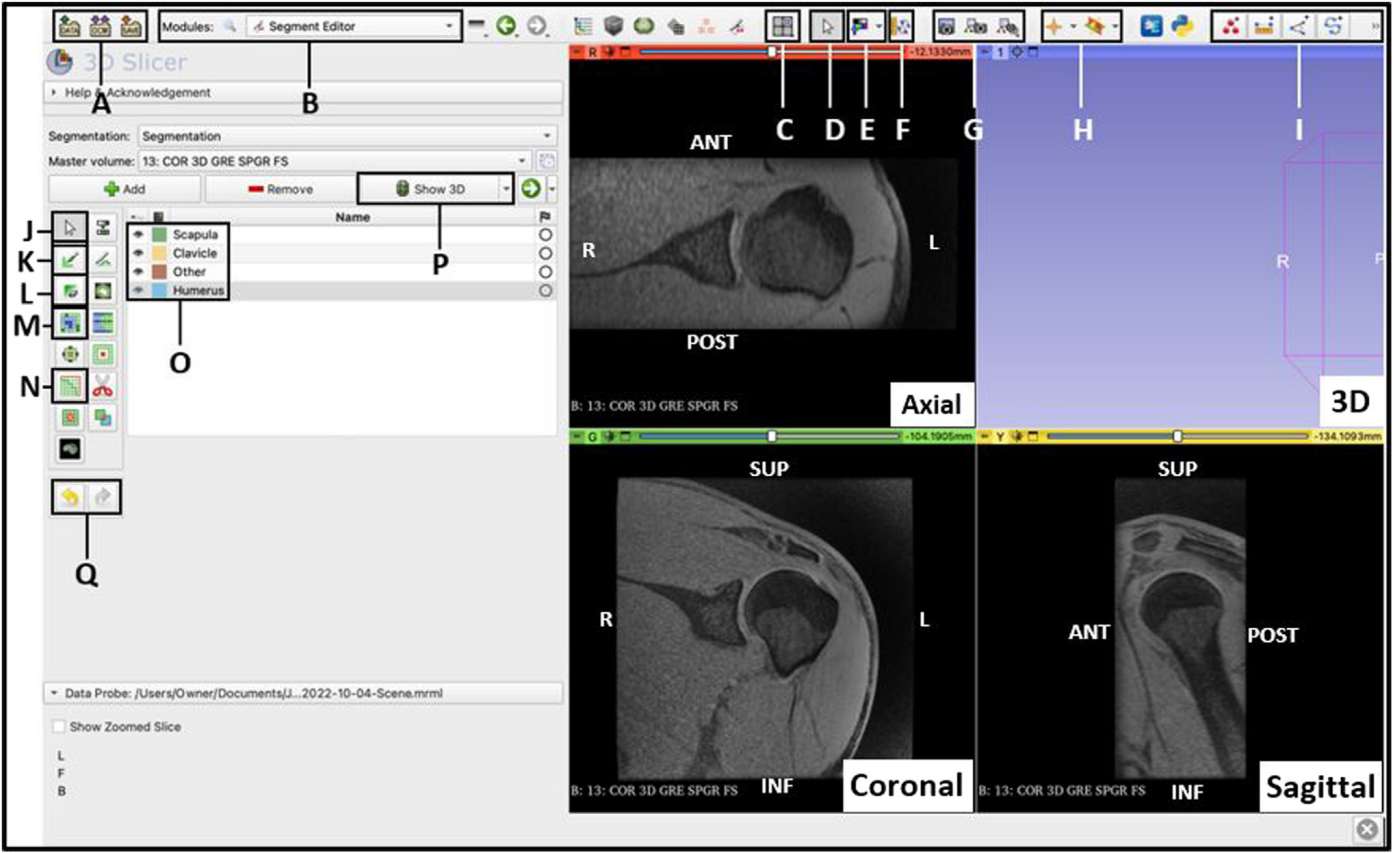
View of the Segment Editor module of the 3D Slicer application, with a patient’s left shoulder 3D spoiled gradient recalled echo functional sequence magnetic resonance imaging scan automatically displayed in the axial, coronal, and sagittal planes. The 3D view is empty as no model has been created yet. Each segment that will be displayed is listed, named, and assigned a color (O). This module provides the tools, functions, and effects that may be used or applied when editing and modifying the segments. Listed are the locations of popular functions used when creating a 3D reconstruction: (A) Load and Save Data; (B) Module List; (C) Change View; (D) Adjust View; (E) Adjust Image Volume; (F) View Markups Toolbar; (G) Record Screenshot of View; (H) View Image Crosshairs; (I) Markups Toolbar (Points, Lines, Angles, Curves, Measurements); (J) No Editing Selection; (K) Paint Effect; (L) Erase Effect; (M) Grow from Seeds Effect; (N) Smoothing Effect; (O) Segment List with Colors and Visibility Option; (P) Show 3D Reconstruction; (Q) Undo and Redo (3D, 3-dimensional; ANT, anterior; INF, inferior; L, left; POST, posterior; R, right; SUP, superior.)
